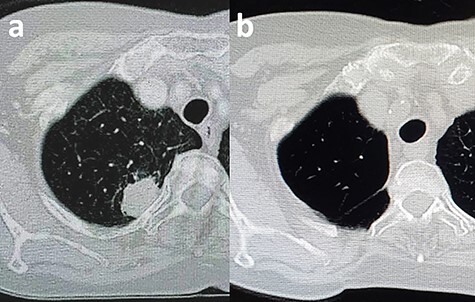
CT scan of the chest. (a) Shown is a tumor located adjacent to the rib’s neck and head. (b) This postoperation image shows the complete resection of the rib’s neck and head.
